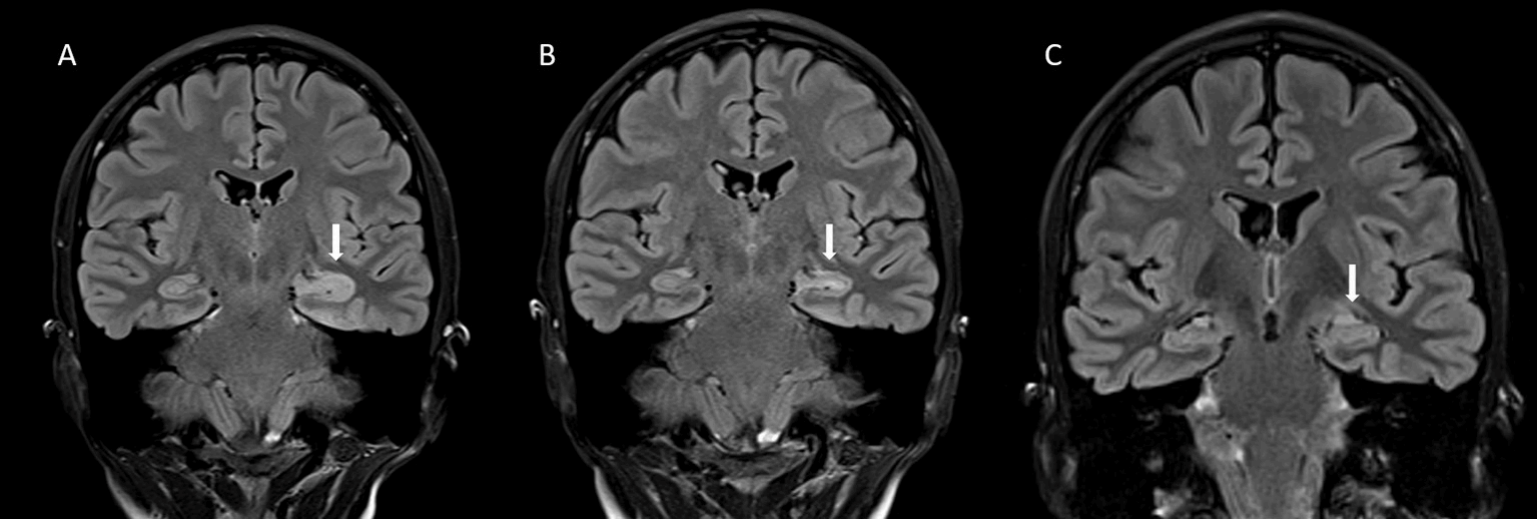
(A) The coronal fluid attenuation inversion recovery (FLAIR) magnetic resonance (MRI) -image taken during the acute stage of the illness shows an abnormally hyperintense and swollen head of the left hippocampus (arrow). (B) Five months later, the finding has mostly resolved, although slight hyperintensity of the left hippocampal head can still be seen (arrow). (C) In a control image, 3 years and 8 months after the acute stage, the abnormal finding has totally resolved (arrow). There are no signs of atrophy in the primarily affected area.
